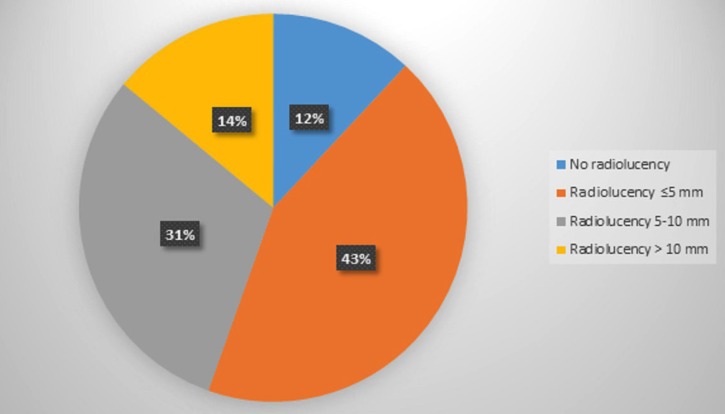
Distruption of the treated teeth according to periapical radiolucency status.
